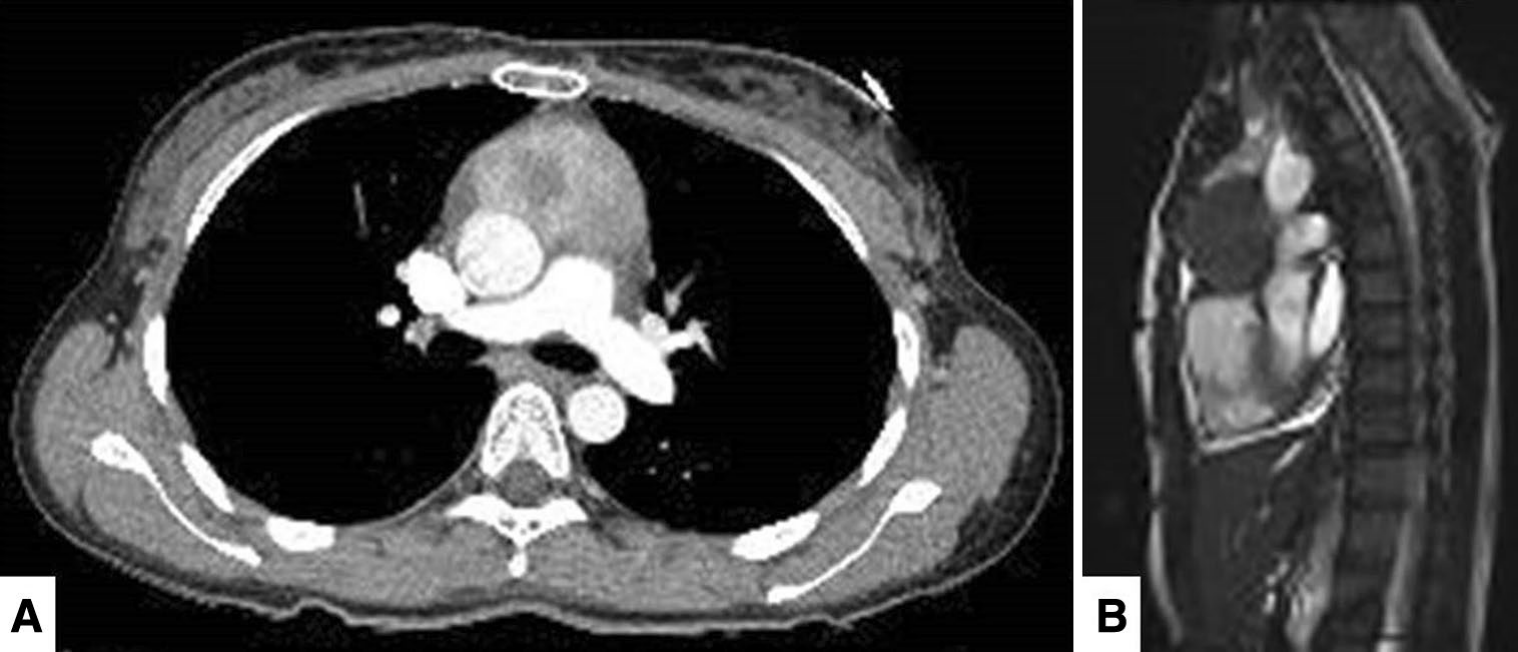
a Chest CT scan (axial section) shows a relatively well-defined heterogeneously enhanced mass in the anterior mediastinum. b Cardiac MRI (sagittal section) shows a mass compressing the ascending aorta and proximal main pulmonary artery. *CT* computed tomography, *MRI* magnetic resonance imaging
